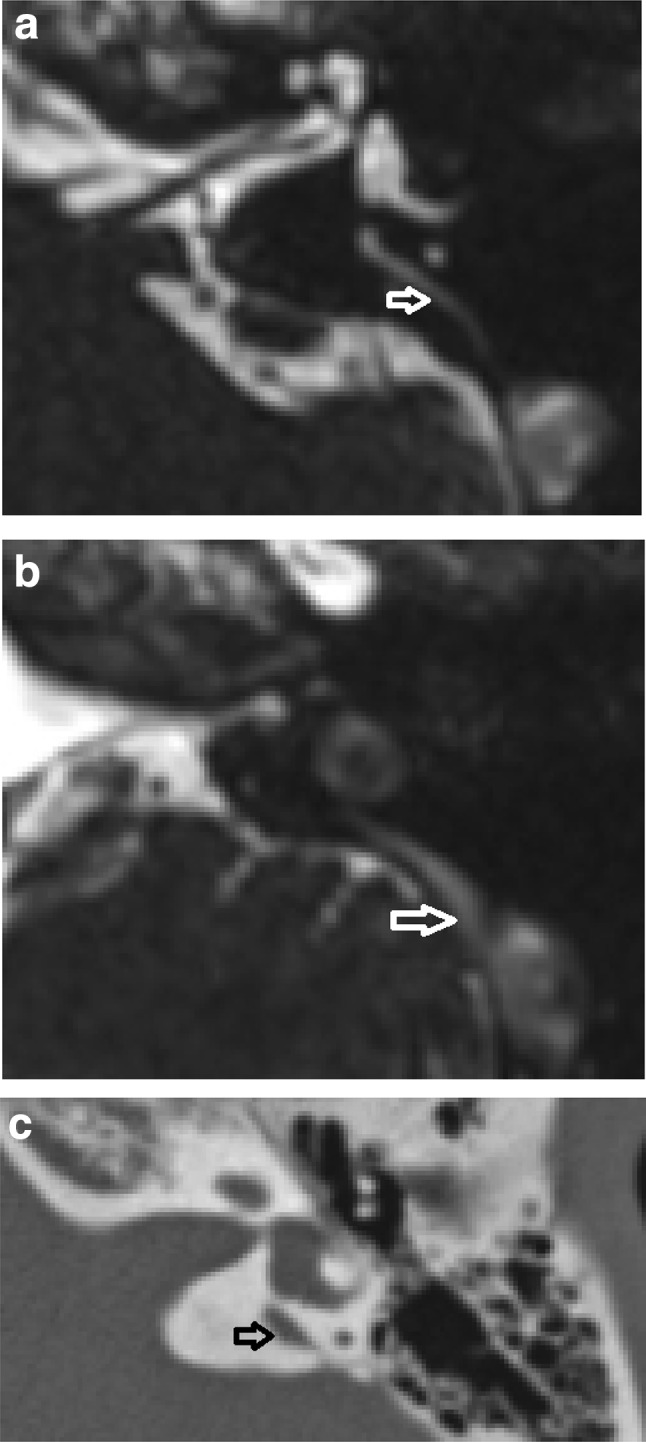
Cincinnati criteria positive but Valvassori criteria negative cases. T2 CISS axial images (a) and (b) demonstrate an elongated intra-osseous endolymphatic sac/duct. The midpoint (white open arrow in a) is not widened on either criteria. At the opercular portion, there is widening (white open arrow in b) so the case is Cincinnati criteria positive but Valvassori criteria negative. Note how it is difficult to define the transition between the bony operculum and the low signal dura overlying the extra-osseous sac. c A different patient demonstrated a minimally widened (1.2 mm) midpoint on CT so the case is also Cincinnati criteria positive but Valvassori criteria negative
